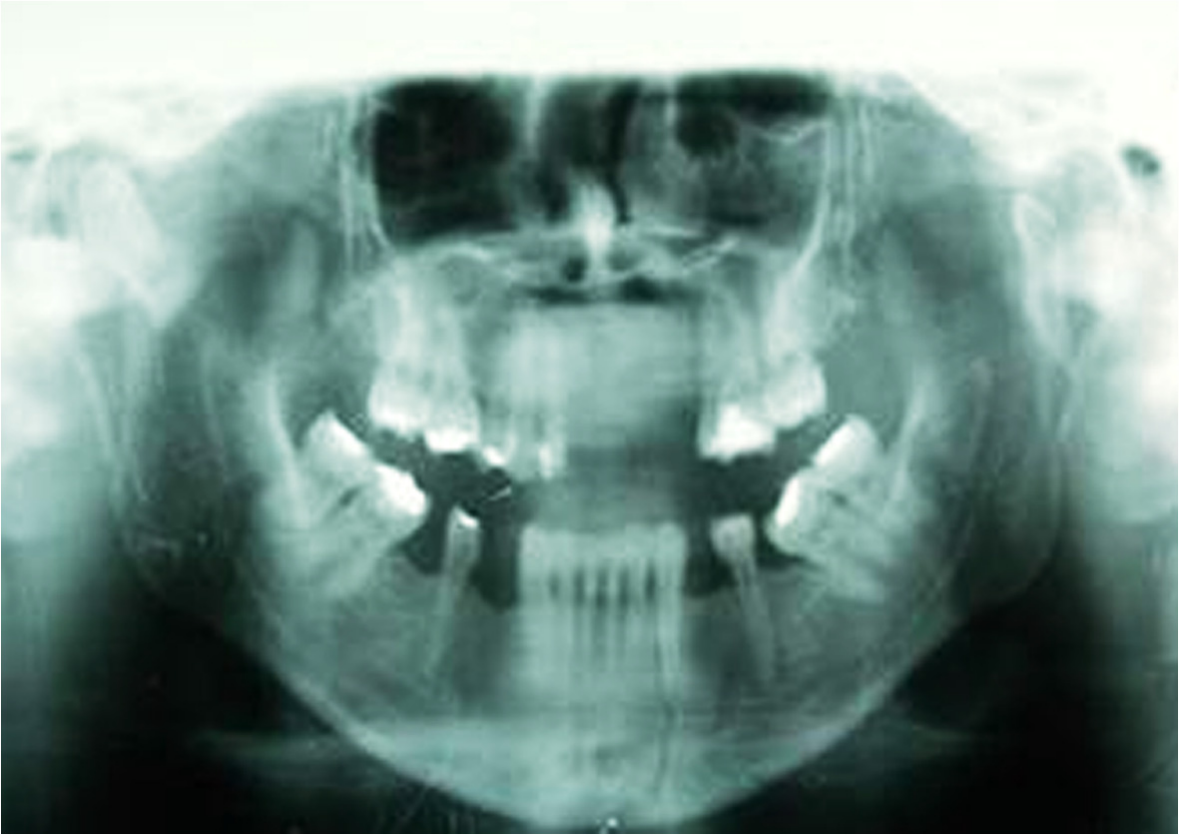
Pre operative Orthopantomograph.
